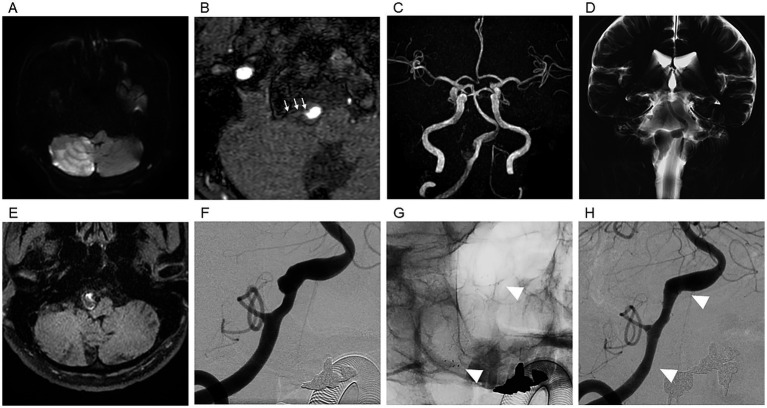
Representative case 1 (48-year-old man). (A) DWI on admission showing acute infarction in the right PICA territory. (B) TOF-MRA demonstrate that the posterior inferior cerebellar artery (PICA) origin was poorly visualized on initial imaging, which limited precise determination of the occlusion site. (C) TOF-MRA demonstrating right V4 dilatation. (D) BPAS showing outer-diameter enlargement. (E) 3D T1-weighted variable flip-angle TSE (T1-CUBE) image showing intraluminal thrombus (white arrow), supporting the diagnosis of a partially thrombosed dissecting aneurysm. (F) Preoperative DSA showing a dissecting aneurysm involving the PICA origin. (G) Fluoroscopic image after FRED placement (arrowheads). (H) Follow-up DSA at 6 months demonstrating adequate occlusion/remodeling (OKM C3). White arrowheads indicate the distal and proximal edges of the flow diverter stent.
